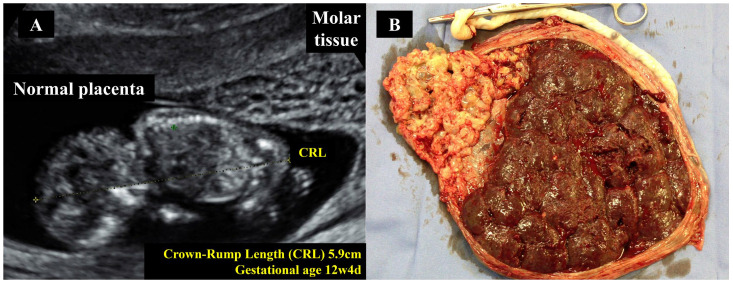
Twin pregnancy with a coexisting complete mole. In panel (A), obstetric ultrasonography at 17 weeks of gestation shows a fetus adjacent to a heterogeneous placenta, exhibiting both areas of normal morphology alongside multiple anechoic regions. These findings suggest a twin pregnancy with a coexisting mole. Fetal karyotyping via amniocentesis confirmed a diploid genome. With close prenatal monitoring, the pregnancy progressed to term and was delivered by cesarean section due to complete placenta previa. The infant weighed 2700 g with Apgar scores of 8 and 9 at one minute and five minutes, respectively. Panel (B) shows the placenta post-delivery, with a distinct molar region confined within otherwise normal placental tissue. The images refer to clinical cases managed by the authors.
